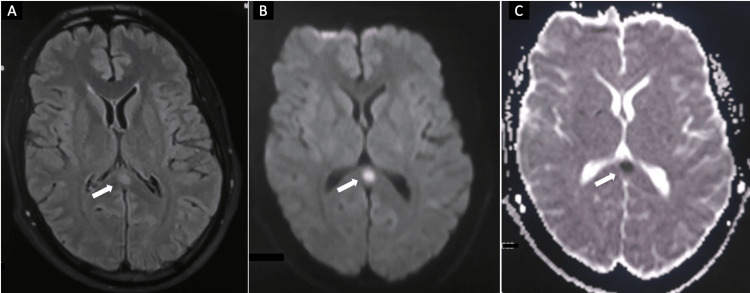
Axial brain MRI of our patient, in the acute phase, showing a lesion in the splenium of the corpus callosum demonstrating hyperintensity in FLAIR sequence (A) with high signal intensity on DWI (B), and markedly low signal intensity on ADC sequence (C). FLAIR: Fluid-attenuated inversion recovery, DWI: Diffusion-weighted imaging, ADC: Apparent diffusion coefficient.
